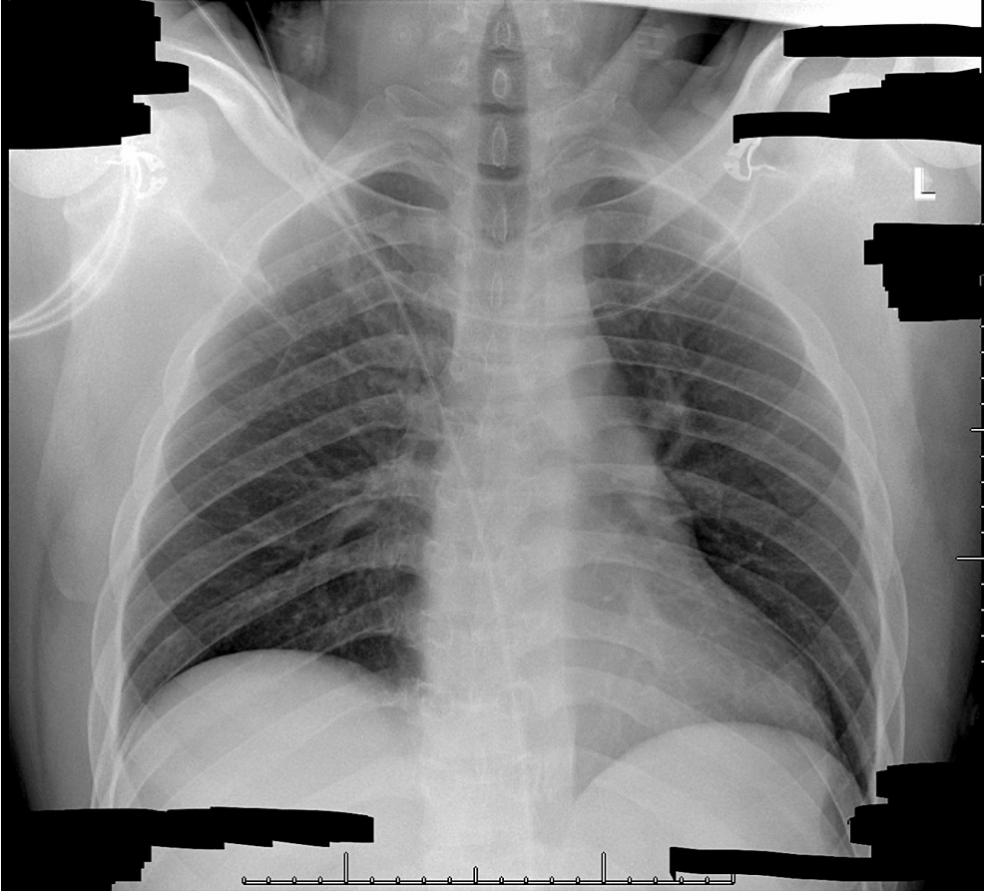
Portable chest radiograph. A single frontal view of the chest was obtained portably. The cardiomediastinal silhouette is within normal limits for technique. There is no focal consolidation, pneumothorax, pulmonary vascular congestion, or sizable pleural effusion. The visualized osseous structures are grossly intact.
